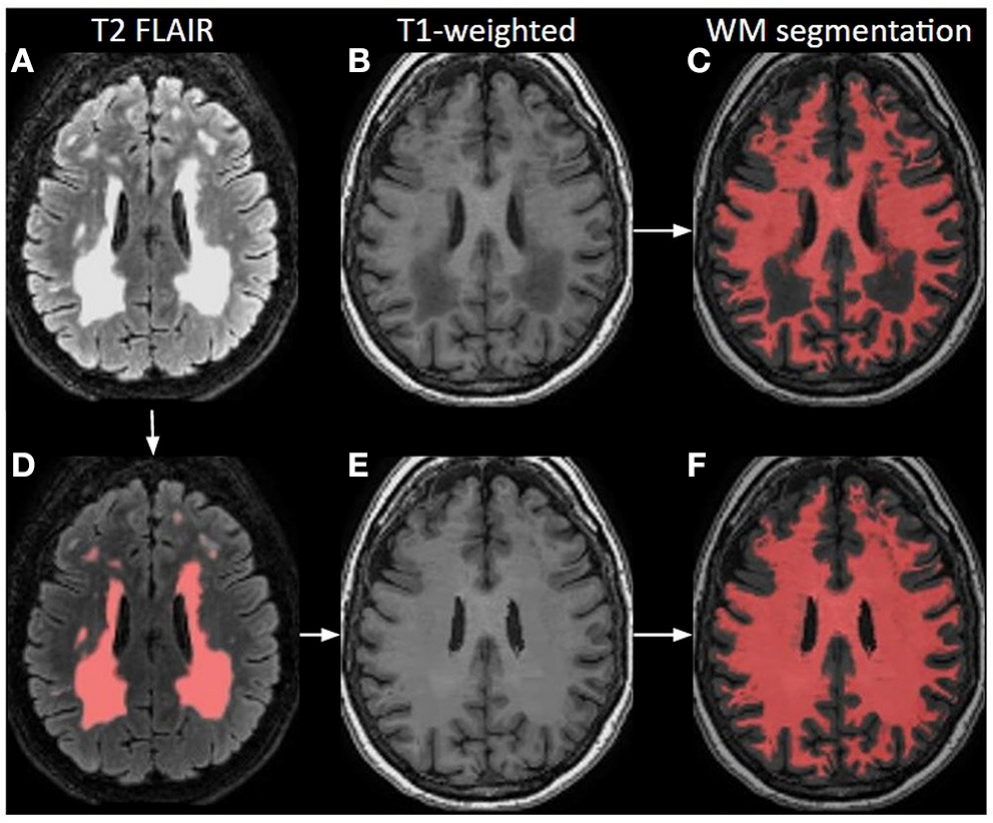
Principle of white matter lesion correction. A T2 FLAIR image (A) with lesion hyperintensities, corresponding to an original T1-weighted image; (B) and a WM segmentation image; (C) from a 70-year-old female. The lesions are automatically identified and segmented on the T2 FLAIR; (D) which are used as a mask to define hypointense regions in T1-weighted images that could be misinterpreted as GM. Based on these lesion masks, the original T1-weighted image is corrected; (E) and shown with the final WM segmentation overlaid in red (F).
